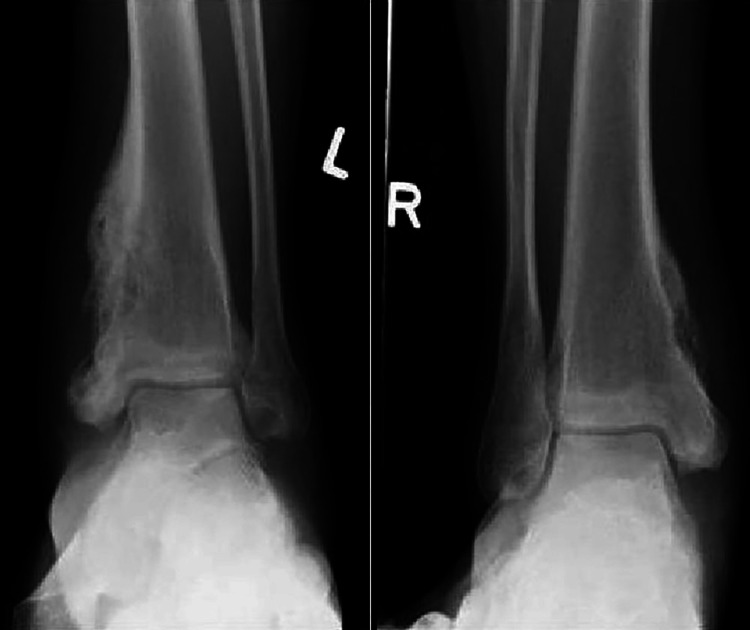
Left and right X-ray of the ankle. There is a periosteal reaction seen along with the medial aspects of bilateral distal tibial meta-diaphysis. This is more florid on the left. There is also sclerosis of the left calcaneus with subtle periosteal reaction at the plantar cortex.
